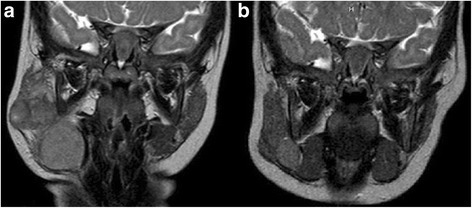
MRI of the face and neck findings. Evidence of a solid mass attributable to lymph nodes, entirely occupying right parotid cavity (a), with marked improvement on second control after prednisone course (b)
